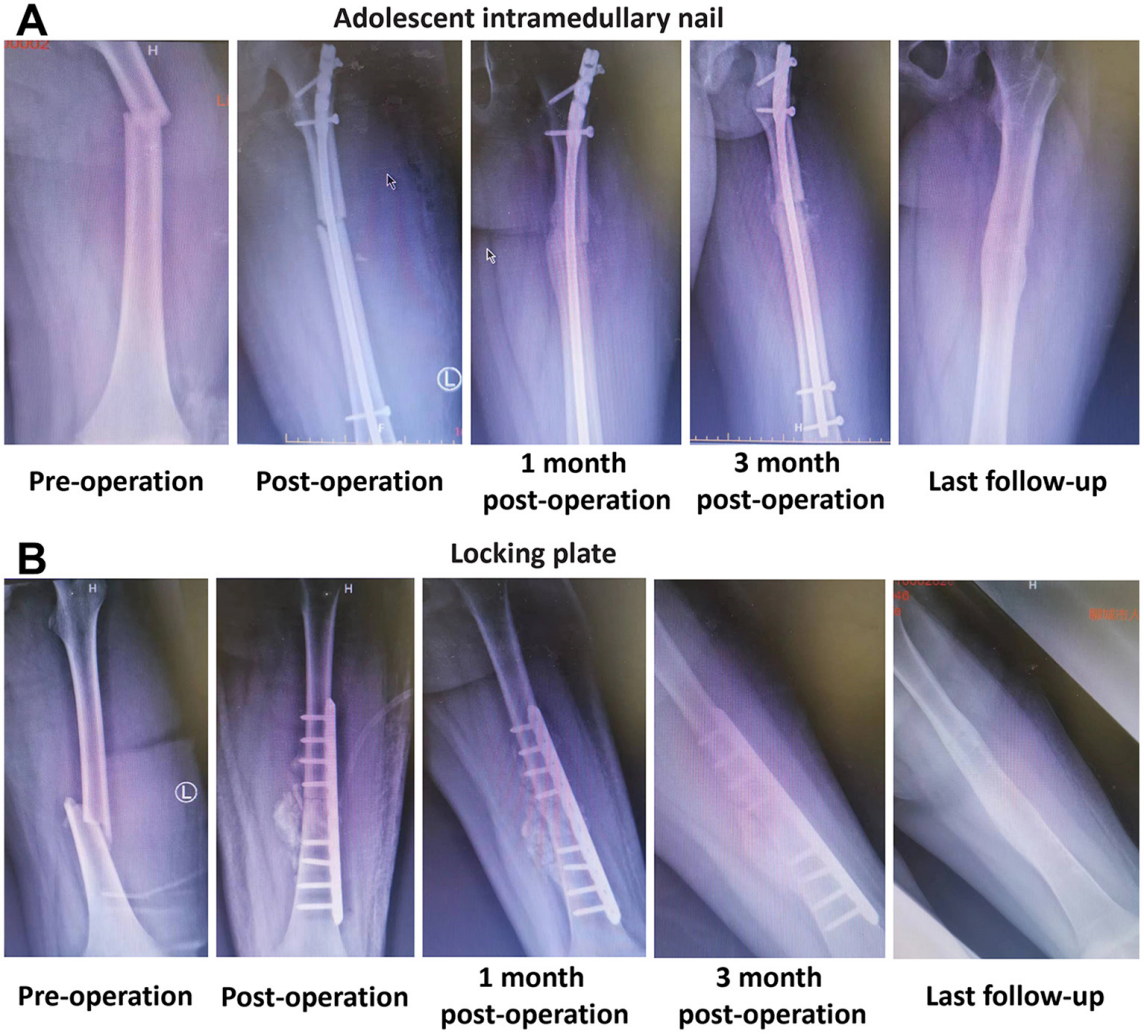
Typical cases of femoral shaft fractures adolescent received the treatment of adolescent intramedullary nail (AIN) or locking plate (LP). (A) X-ray images of a 13 years old boy with left femoral shaft fracture received the treatment of AIN at different times. (B) X-ray images of a 14 years old boy with left femoral shaft fracture received the treatment of LP at different times.
